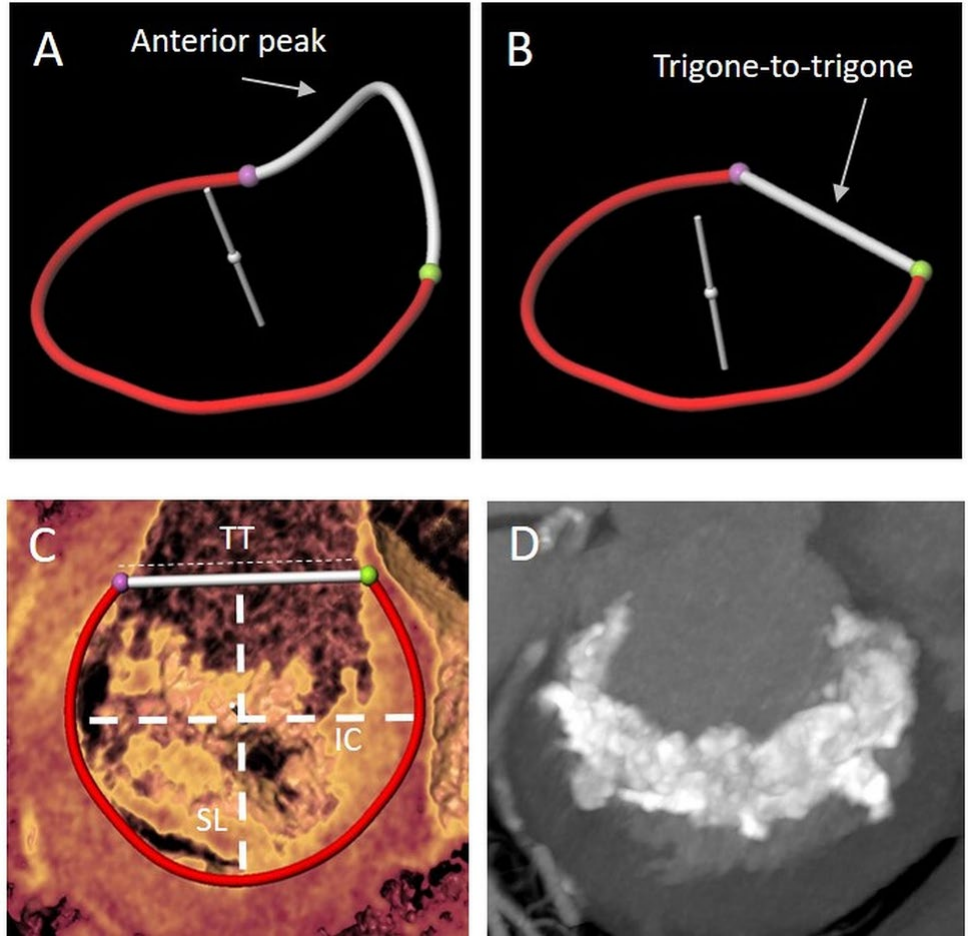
CT for planning transcatheter mitral valve procedures. A–C Mitral annular segmentation (pink dot = medial fibrous trigone; green dot = lateral fibrous trigone) using a semiautomated software approach. A Saddle-shaped mitral annulus with the anterior horn. B The “D-shape” mitral annulus is created by truncating the anterior horn at the trigones resulting in a more planar geometry. C Determination of annular measurements for preprocedural planning (IC, intercommissural distance; SL, septal-lateral distance; TT, trigone-trigone distance). D Short-axis maximum intensity projection showing severe mitral annular calcification
